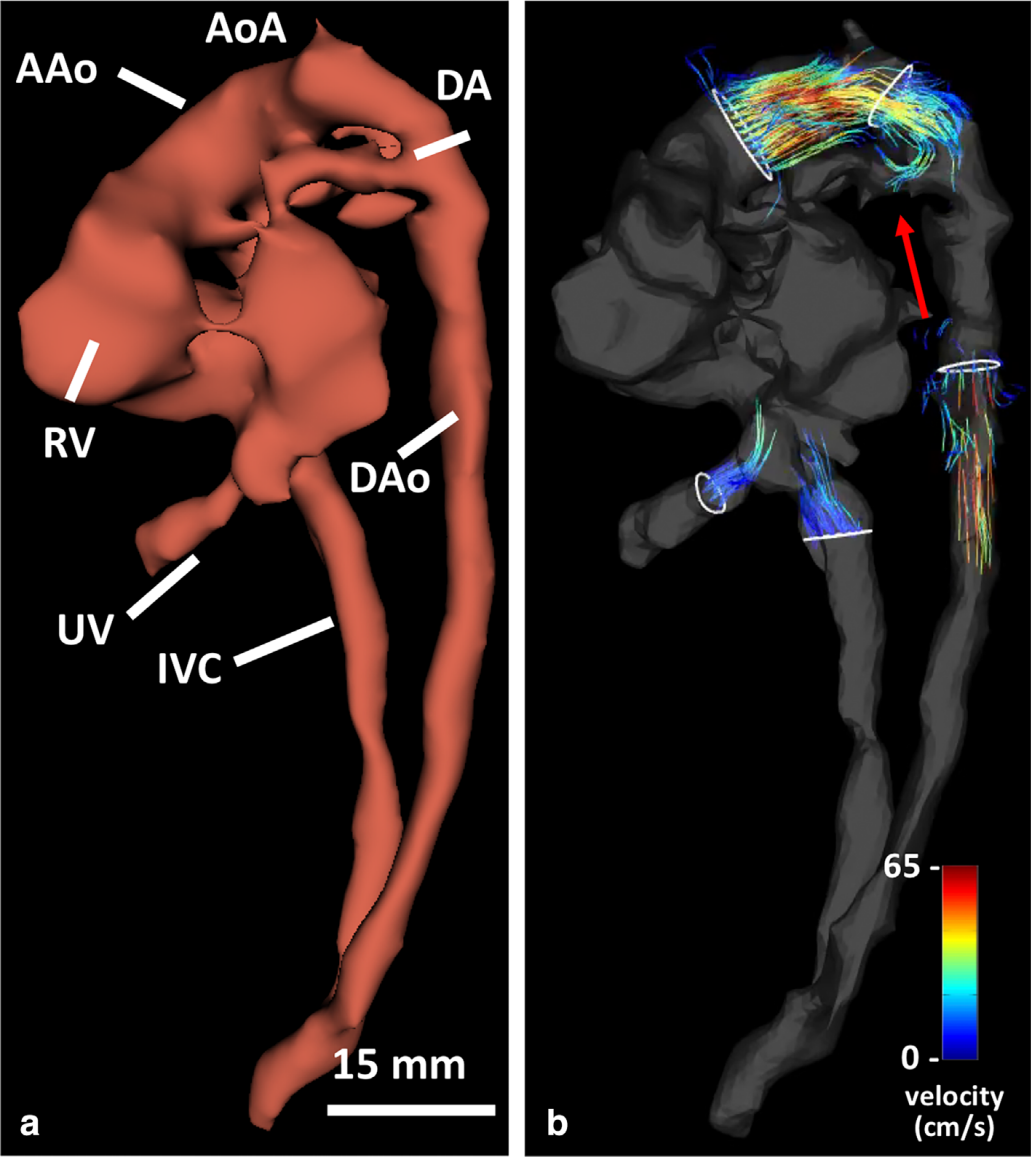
Four‐dimensional (4D) flow fetal cardiovascular MRI in a fetus with transposition of the great arteries. (a) 3D segmentation of fetal vasculature from the 4D‐flow derived phase contrast angiogram. (b) Time‐resolved pathlines (shown at 128 msec from the cardiac trigger) emitted from the denoted vessels, with visible diastolic reverse ductal flow (red arrow). Note the caliber of the aortic arch and descending aorta, helpful to exclude interruption or coarctation. An animated version of these pathlines can be found in Movie S2 in the Supplemental Material. AAo = ascending aorta; AoA = aortic arch; DA = ductus arteriosus; DAo = descending aorta; IVC = inferior vena cava; MPA = main pulmonary artery; RV = right ventricle; UV = umbilical vein.
